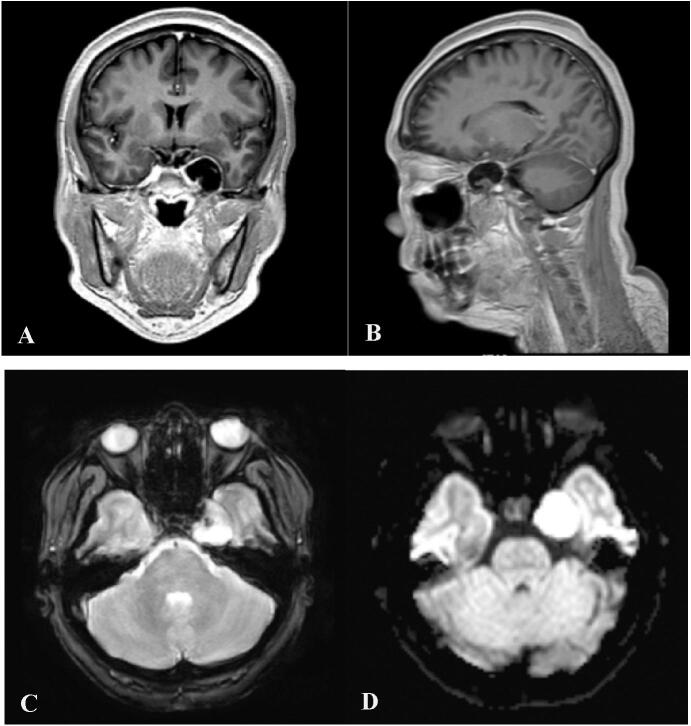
Brain MRI Images reveal an extra-axial mass in the left parasellar region. (A) T1-weighted image showing hypointense signal, (B) Sagittal view of the parasellar bones does not show any signs of infiltration from the mass (B) T2-weighted images showing hyperintense signal that is isointense to CSF, (C) DWI image showing hyperintense signal.
